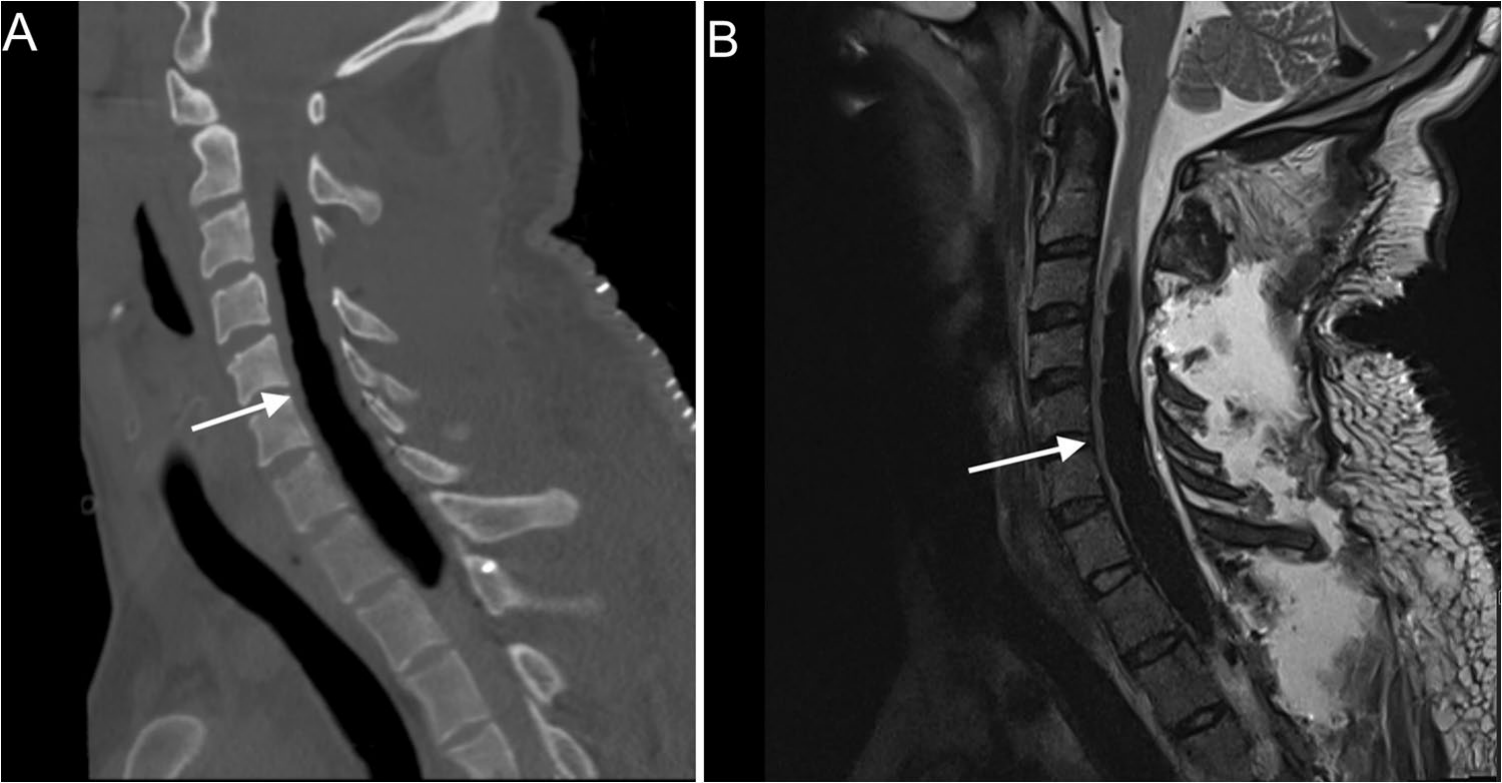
A Post-operative cervical sagittal CT demonstrating a tension pneumosyrinx extending from C3 to the level of T2; B Post-operative sagittal T2-weighted MRI of the cervical spine demonstrating a hypointense lesion at the same level which is consistent with a tension pneumosyrinx
